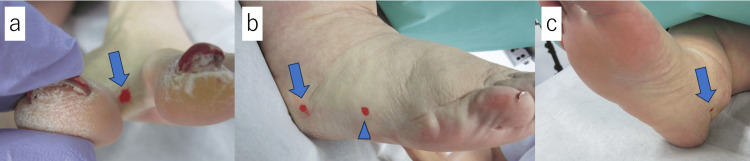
The injection points in the LyS4 examination. a–c: The injection points in the LyS4 examination. Technetium-99m human serum albumin diethylenetriamine pentaacetic acid (^99m^Tc-HSA-DTPA) is injected at the first interdigital web (a: arrow), slightly below the area of the lateral malleolus (b: arrow), the lateral side of the feet near the fifth digit (b: arrowhead) and slightly below the area of the medial malleolus (c: arrow) and of both feet before the image acquisition. LyS4: four-point dermal injection lymphatic scintigraphy
